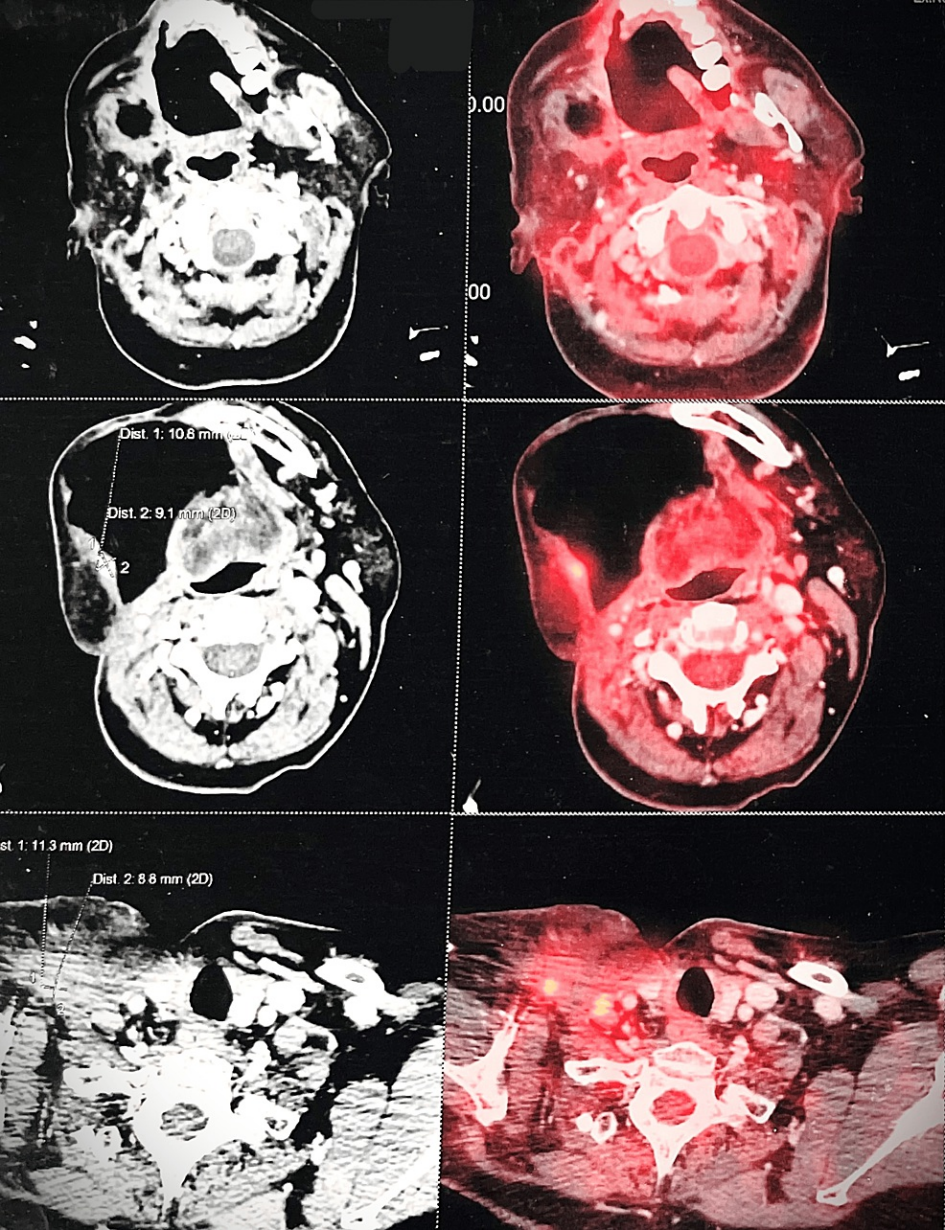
Images shows no evidence of metabolically active lesion of hard palate, as compared to previous PET-CT image shows reduction in size and metabolic activity of enhancing lesion in postero-lateral margin of flap-inflammatory. Reduction in size and metabolic activity of ill-defined enhancing lesion in right supraclavicular region - likely inflammatory.
